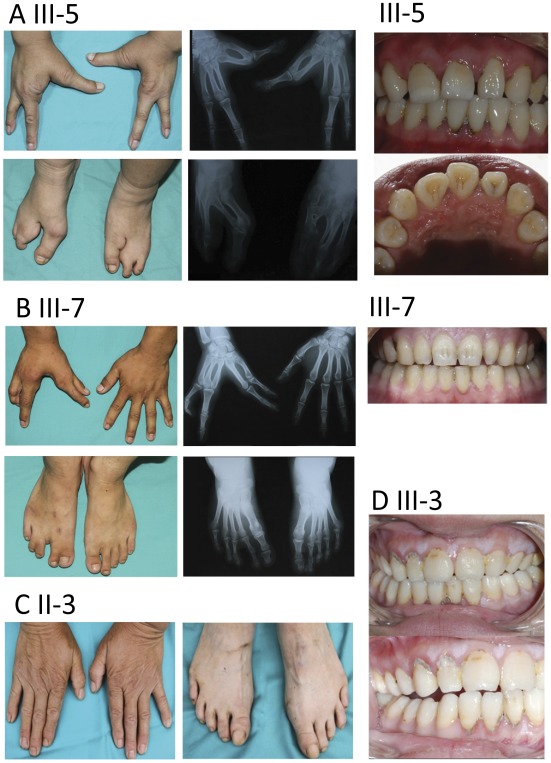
Three types of hands/feet manifestations and malformed teeth. (A) Symmetrical deformities. Patient III-5: absence of 2nd–3rd fingers and 2nd–3rd toes. Hand radiography: absence of 2nd–3rd phalanges and fusions of the 1st–2nd and 3rd–4th metacarpale in both hands. Feet radiography: absence of 2nd–3rd phalanx and osseous fusion of 1st–2nd metatarsal bone. Left panel: spade-shaped central incisor and open bite. (B) Asymmetric deformities. Patient III-7: Syndactyly of 1st–2nd finger, absence of middle finger, syndactyly of 1st–2nd and 3rd–4th toes. Radiography: missing third phalanges and cutaneous syndactyly of 1st–2nd and 3rd–4th toes. Left panel: cone-shaped left maxillary lateral incisor. (C) Patient II-3: Hands or feet were undistinguished from normal controls. (D) Patient III-3: Absent right maxillary lateral incisor and malformed lingual fossa in the left maxillary lateral incisor.
